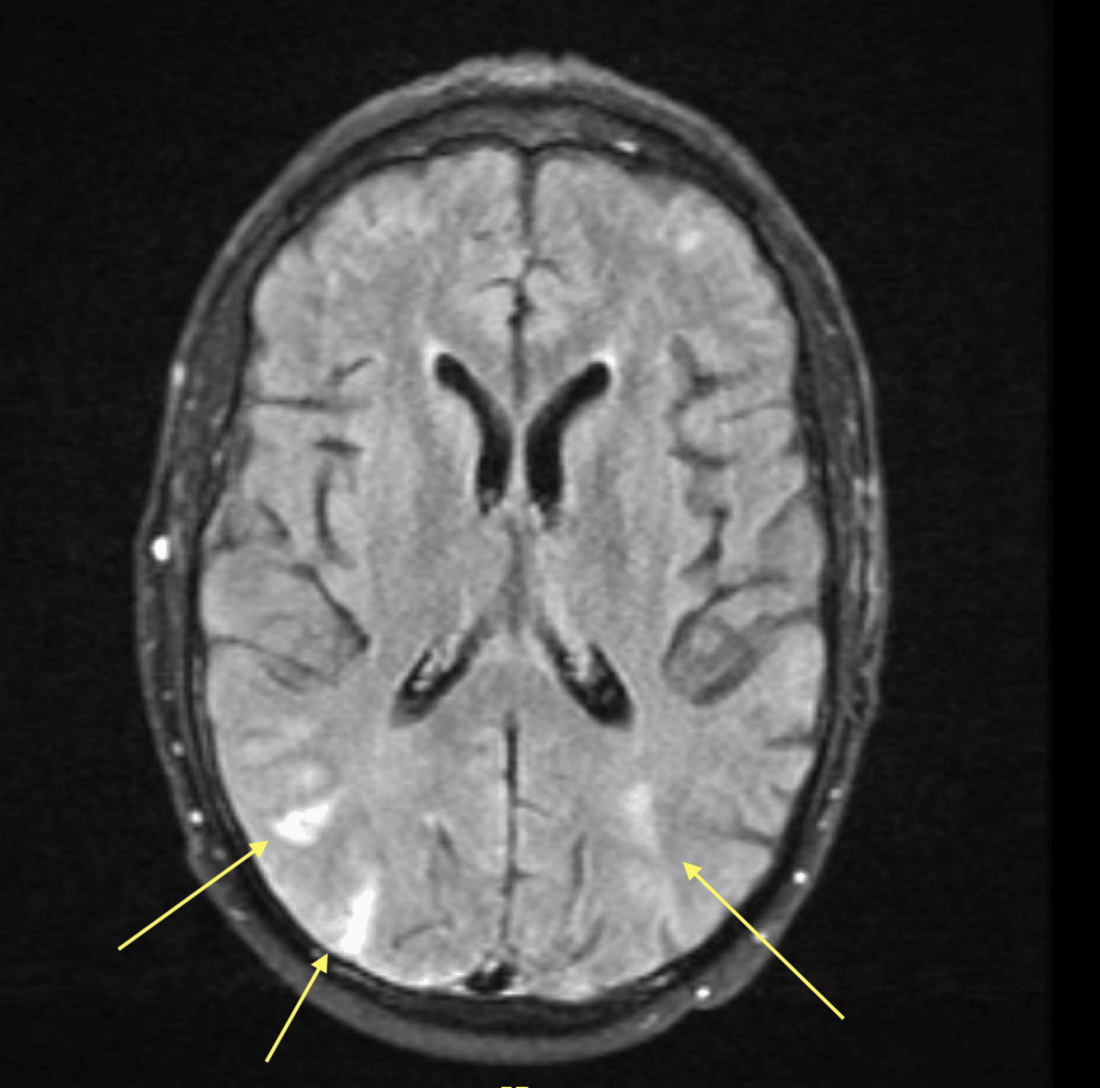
Magnetic resonance imaging of the brain during a code stroke demonstrating bilateral posterior fluid-attenuated inversion recovery (FLAIR) hyperintensities
